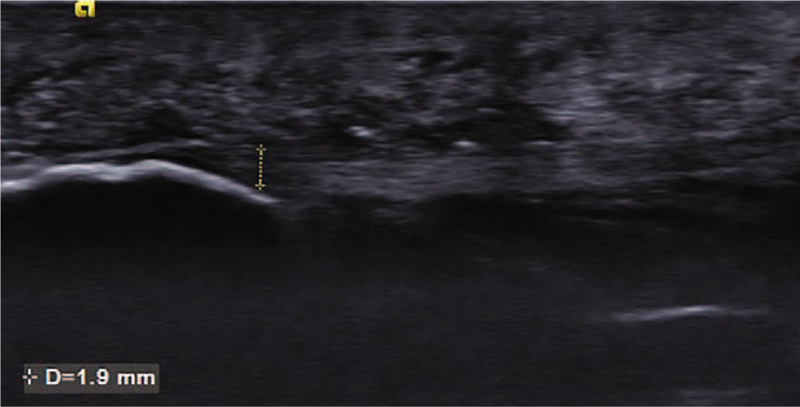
Musculoskeletal ultrasound showed that both the thickness of plantar fascia and the fibrillar structure were normal.
